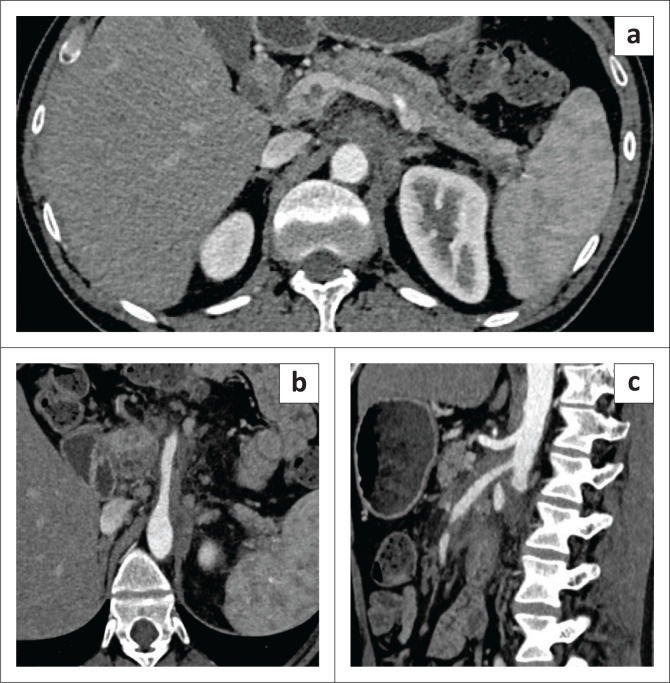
Axial (a–b) and sagittal (c) sections of the CT abdomen of case 3 reveal features of chronic pancreatitis (a), soft tissue cuffing of the long segment of the superior mesenteric artery (b–c).
